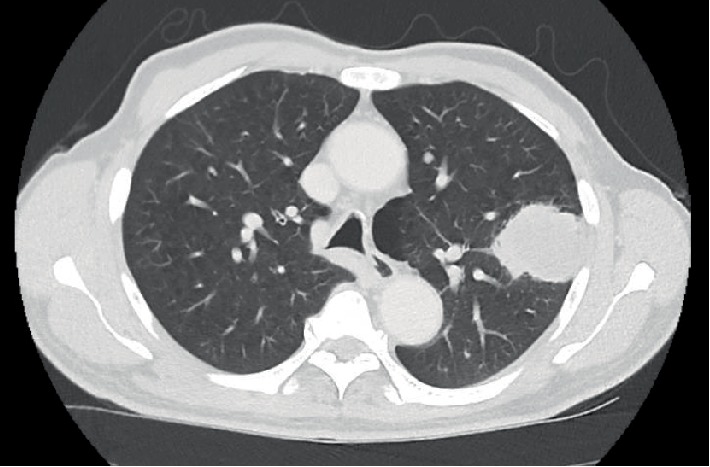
CT scan (axial) showing a 4.3 × 3.4 cm spiculated hyperechoic lesion in the left upper lobe abutting the pleura.
